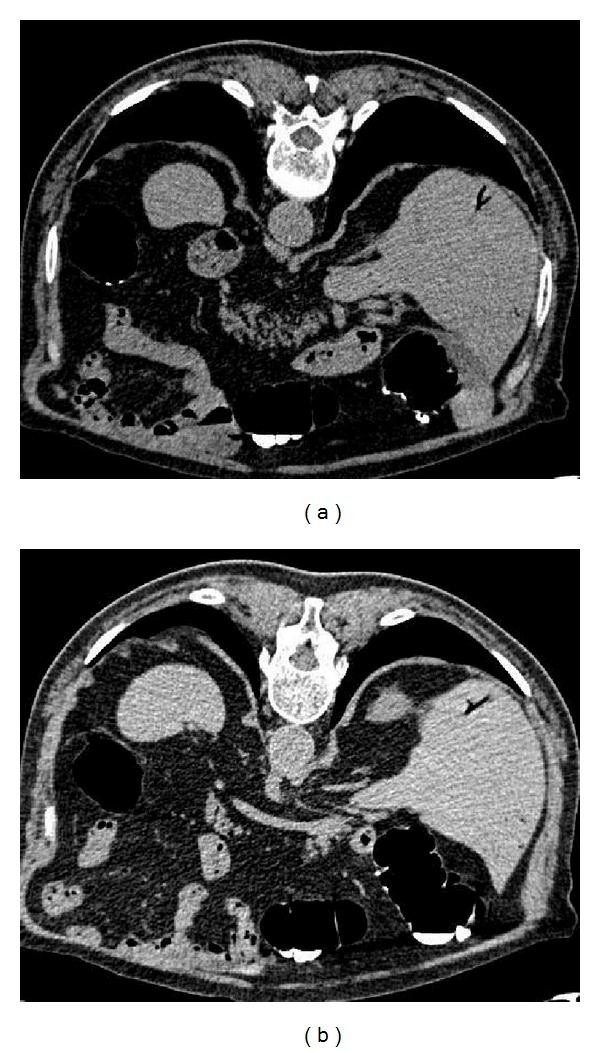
(a) Transverse CT scans revealed the presence of gas in both hepatic lobes. (b) Transverse CT scans revealed the presence of gas in both hepatic lobes.
